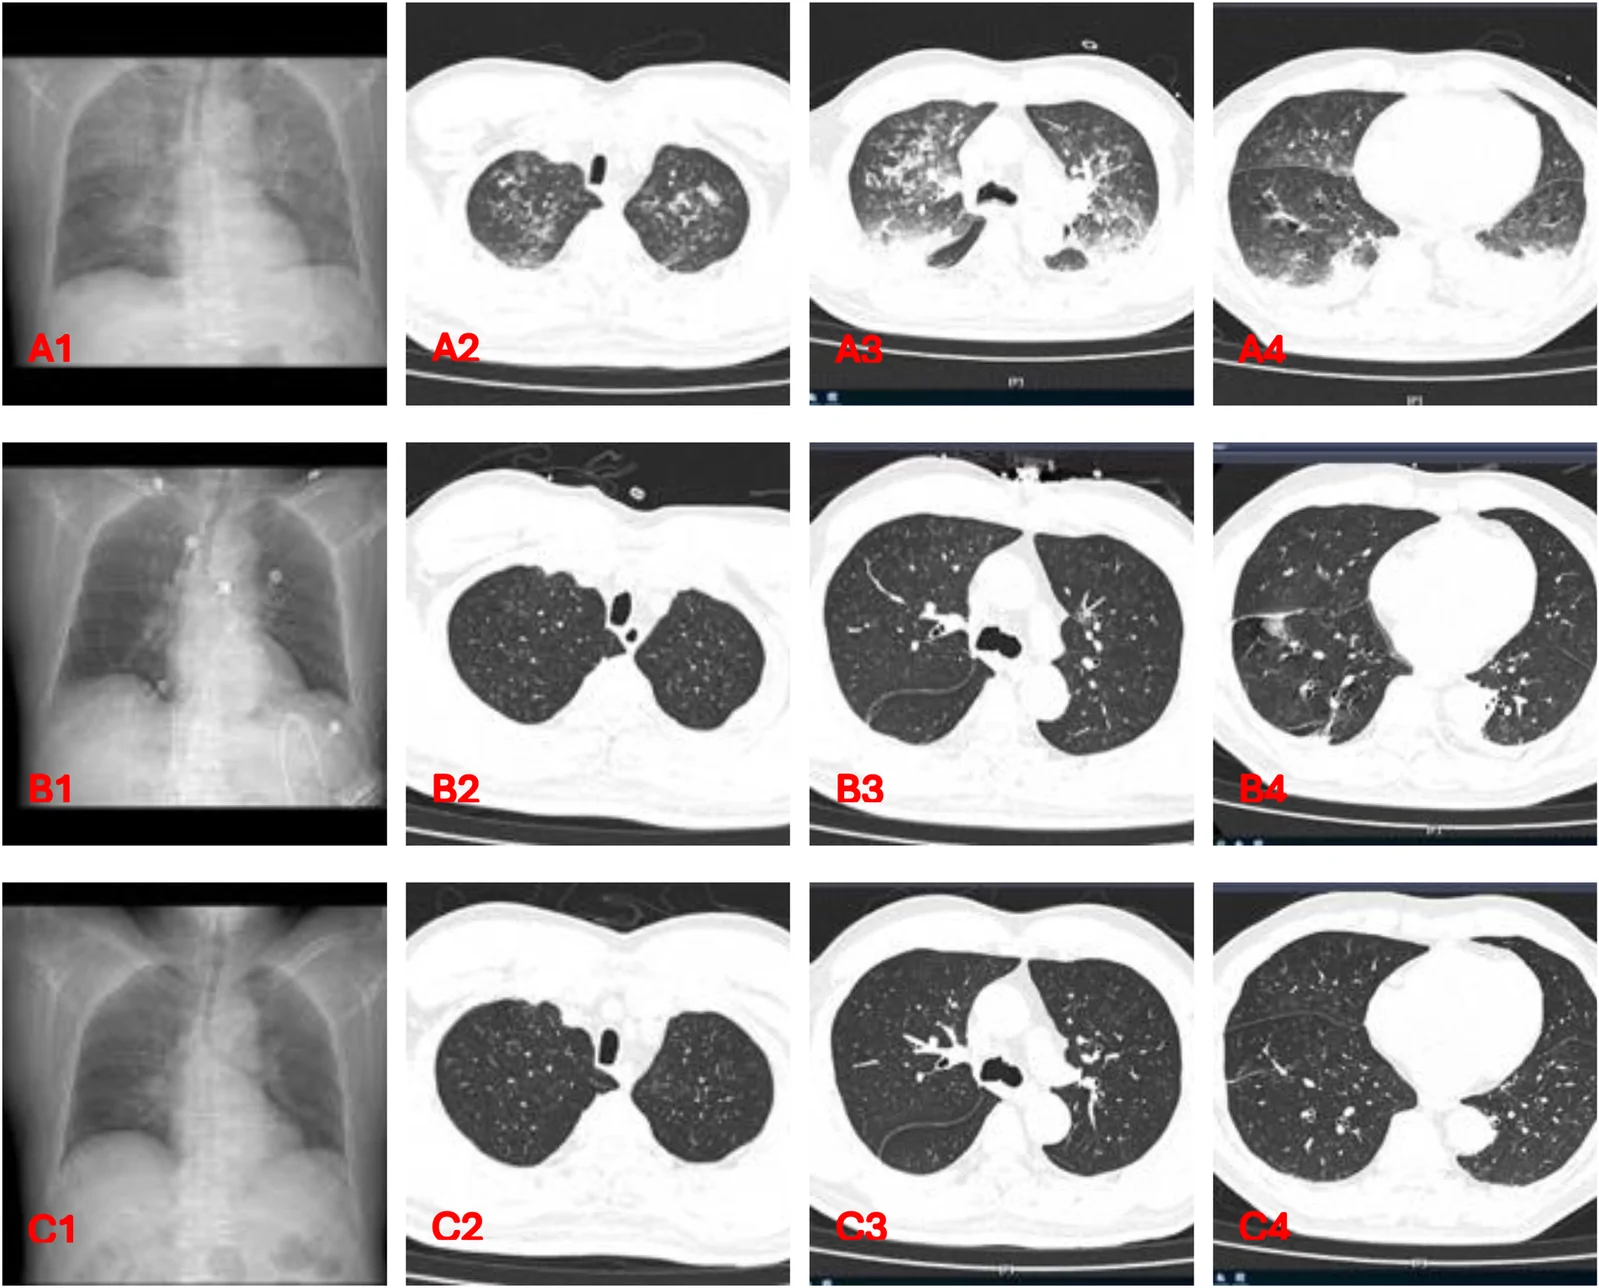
Chest CT findings after treatment for chlorine inhalation–induced ARDS. (A1–C1) Chest x-ray on Day 4, Day 10, and Day 26. (A1–A4) Day 4 imaging demonstrated diffuse bilateral ground-glass opacities and patchy consolidations, predominantly in the dependent and lower lung regions. (B1–B4) Day 10 imaging showed marked regression of pulmonary opacities with improved lung aeration. (C1–C4) Day 26 imaging revealed near-complete resolution of the previous abnormalities, indicating substantial recovery of chlorine inhalation–induced lung injury.
